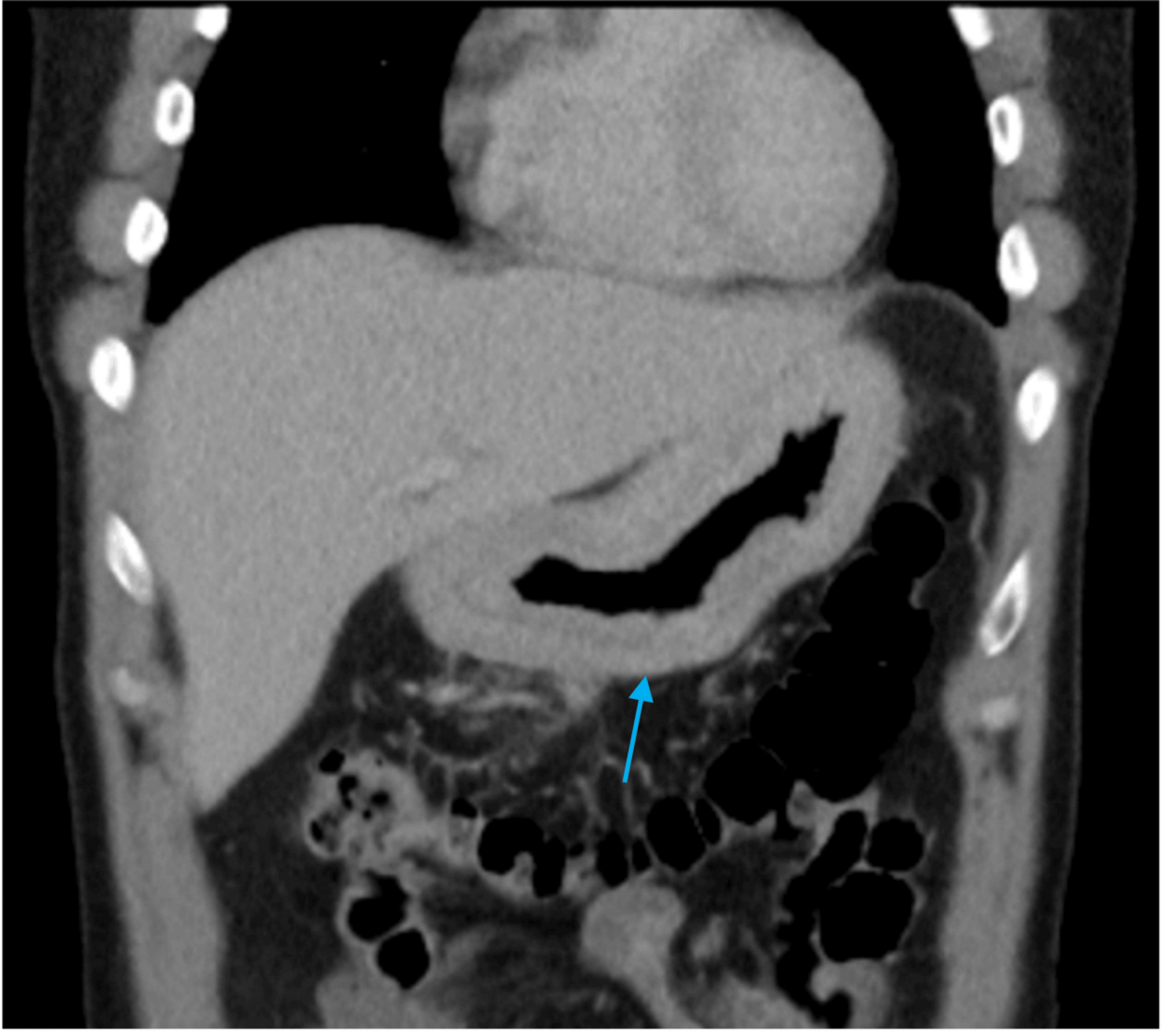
Computed tomography of the abdomen with findings of diffuse gastric wall thickening (blue arrow).
